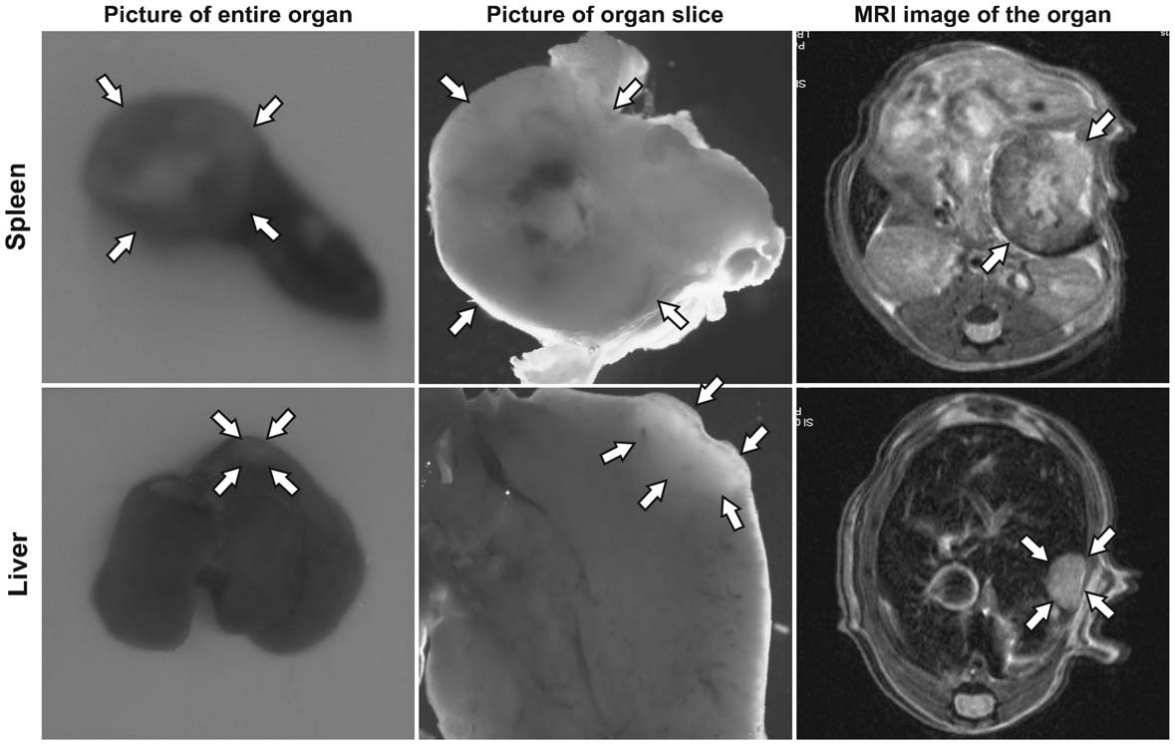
Visualization of primary tumor in the spleen and liver metastasis. Laparotomy observations of the entire organs and sections of the organs where the tumors are engrafted give an accurate idea of the location and shape of the spleen and liver tumors within the organ, which corresponds to its predicted location and shape by MRI.
